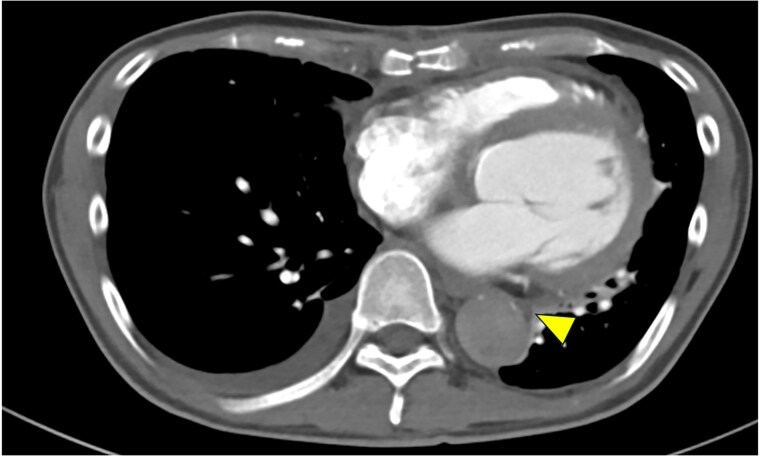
Contrast-enhanced axial computed tomography showing marked narrowing of the true lumen of the descending aorta (yellow arrowhead) due to false lumen expansion.
